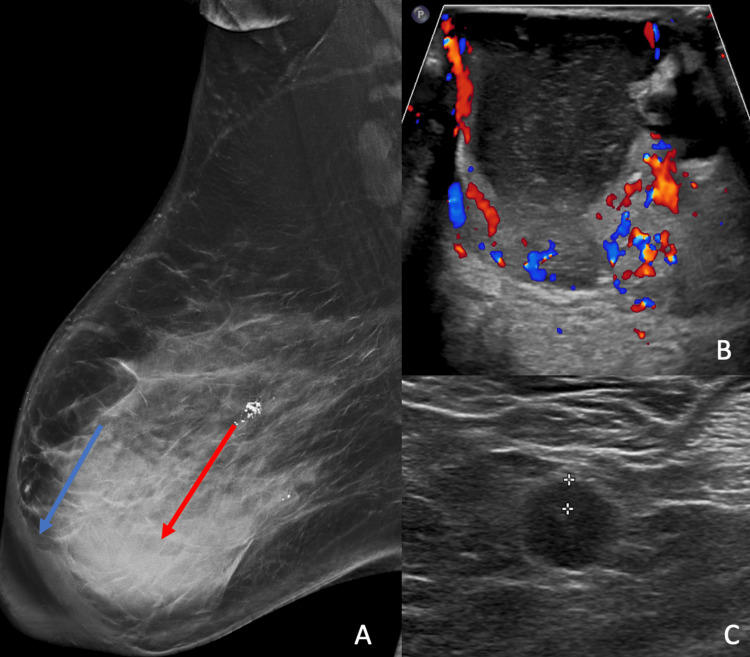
A: Diagnostic mammogram shows a retroareolar right breast mass (red arrow) and associated skin and trabecular thickening (blue arrow); B: Diagnostic ultrasound revealed a hypervascular, complex cystic mass; C: Prominent lymph nodes with concentric cortical thickening, measuring up to 0.4 cm, in the ipsilateral axilla were also found
